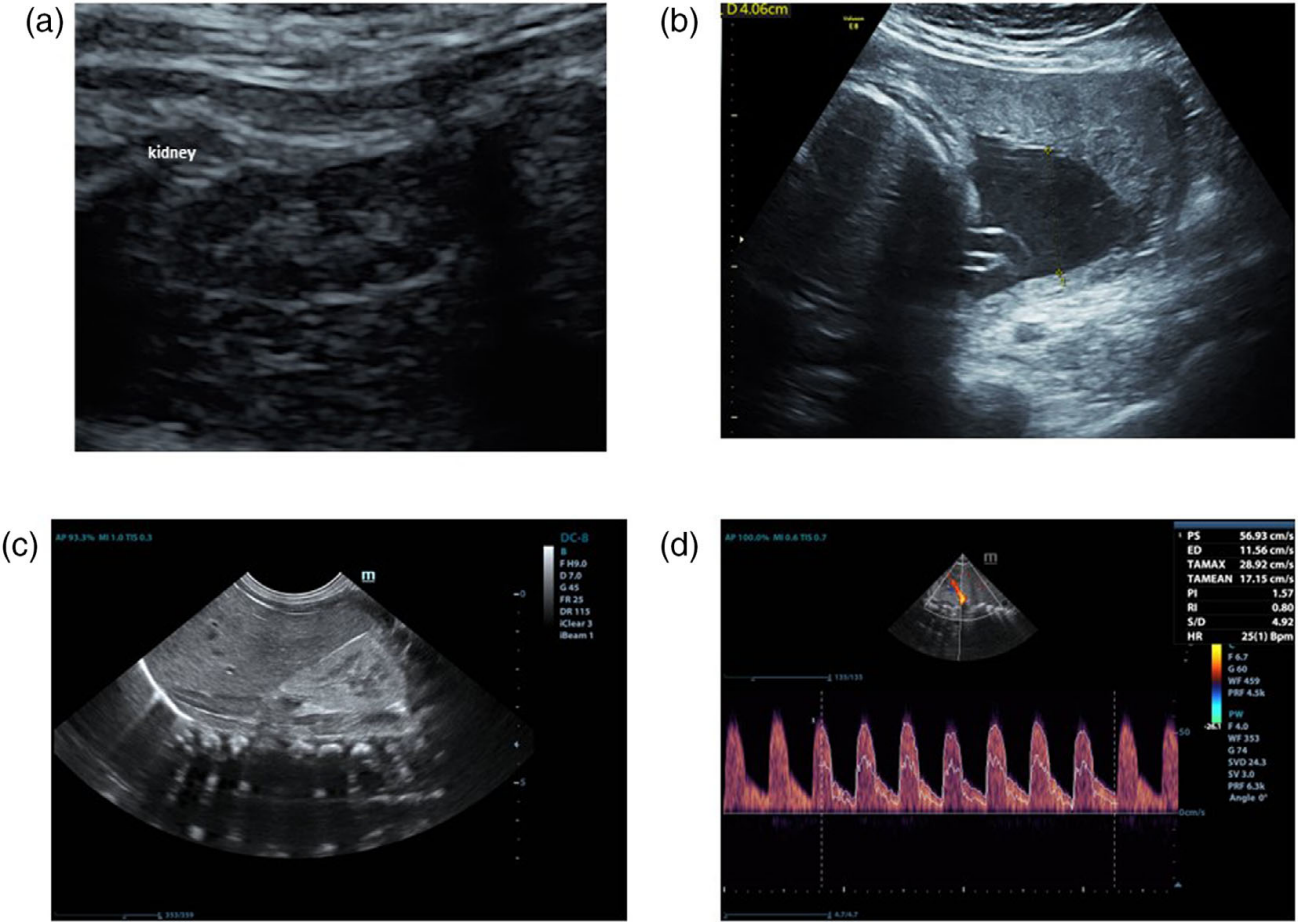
(a) Marked reduction of the amniotic fluid (oligo/anhydramnios) observed at 31 weeks. Normal echogenicity of fetal kidneys. (b) Reappearing of adequate amniotic fluid amount, after maternal therapy suspension, at 32 weeks +5. (c) Ultrasonography of the right neonatal kidney on 3rd day of life showing diffuse increased cortical echogenicity. (d) Mild increase of resistive index in the right renal artery.
